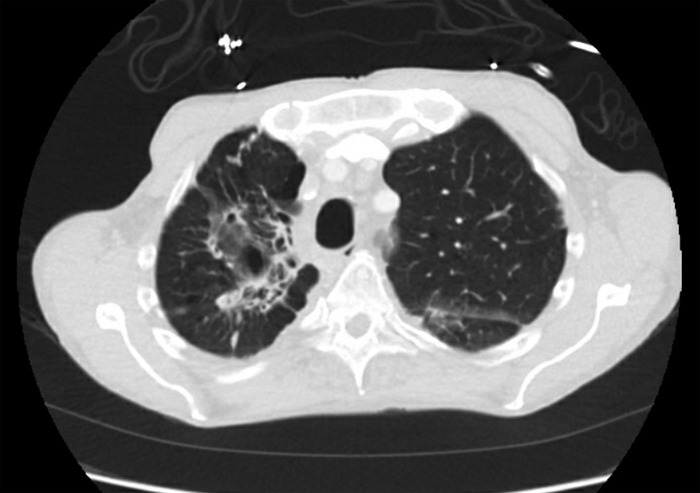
Follow-up chest computed tomography after 5 weeks, transverse view of upper lobes, shows the mural nodule in the right upper lobe intracavitary lesion at 3.1 cm and partially calcified.
